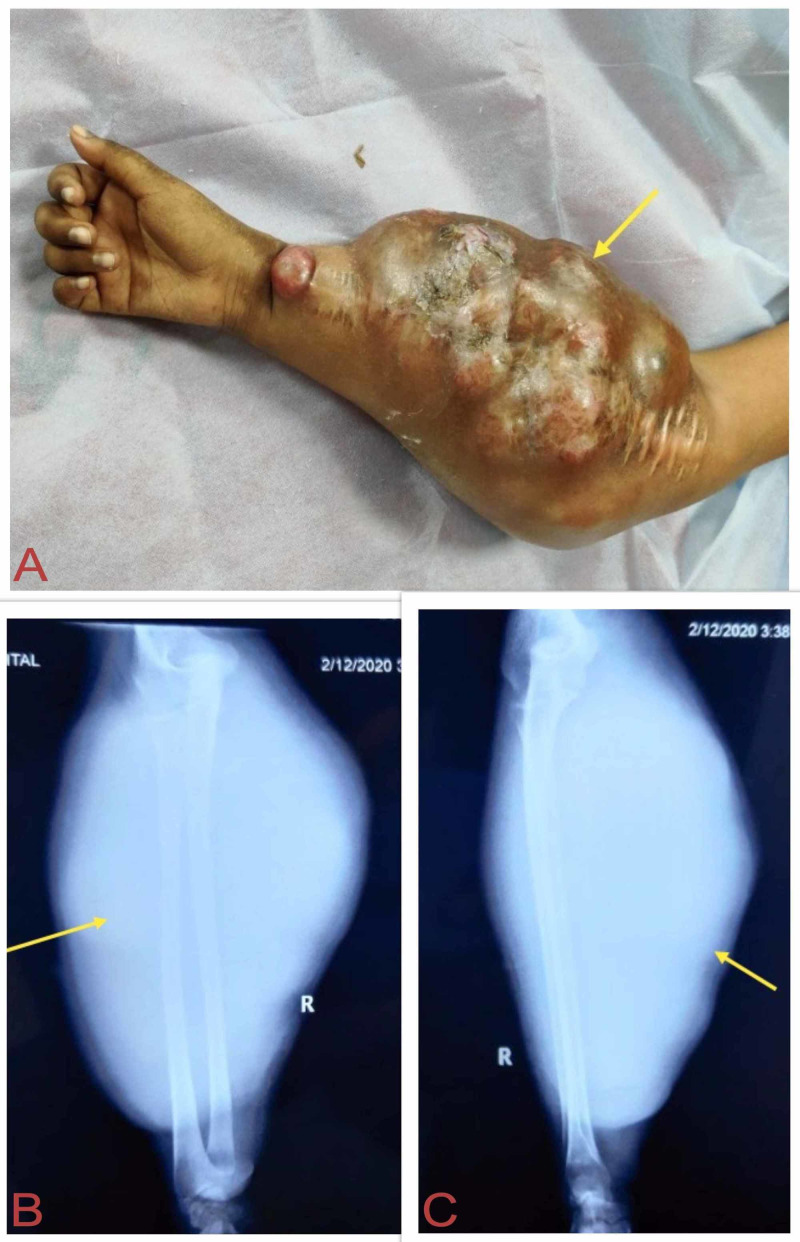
Recurrence after six months. (A) Fungating mass involving the whole of right forearm. (B, C) Anteroposterior and lateral view X-rays show massively increased soft tissue shadows.
